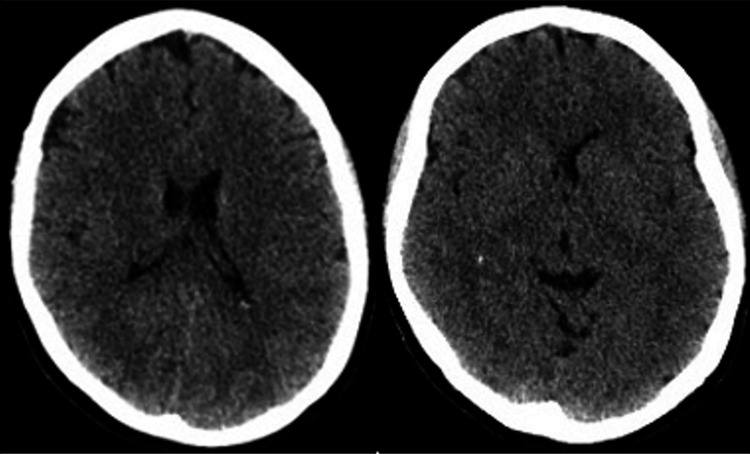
Non-contrast brain tomography of the brain The ventricular system is normal and there is no ischemic area. The Alberta Stroke Programme Early CT Score (ASPECTS) is 10/10.
